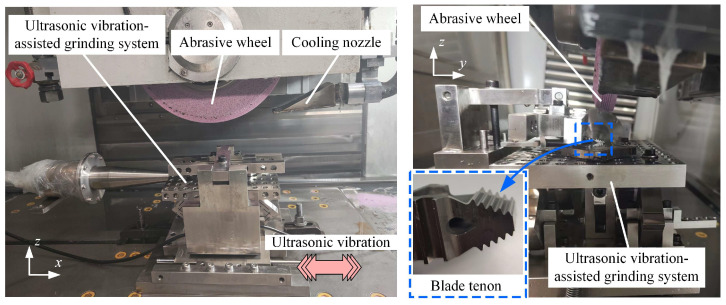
Ultrasonic vibration-assisted grinding experimental device.
